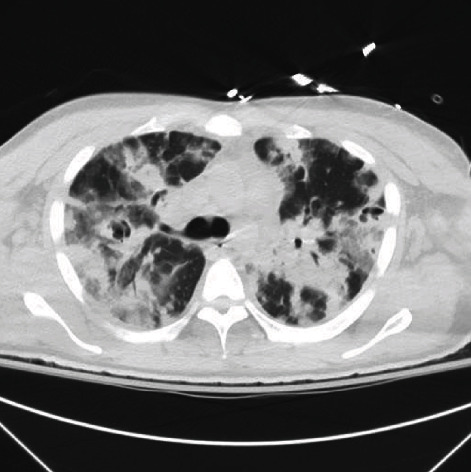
Initial CT chest without contrast findings.
